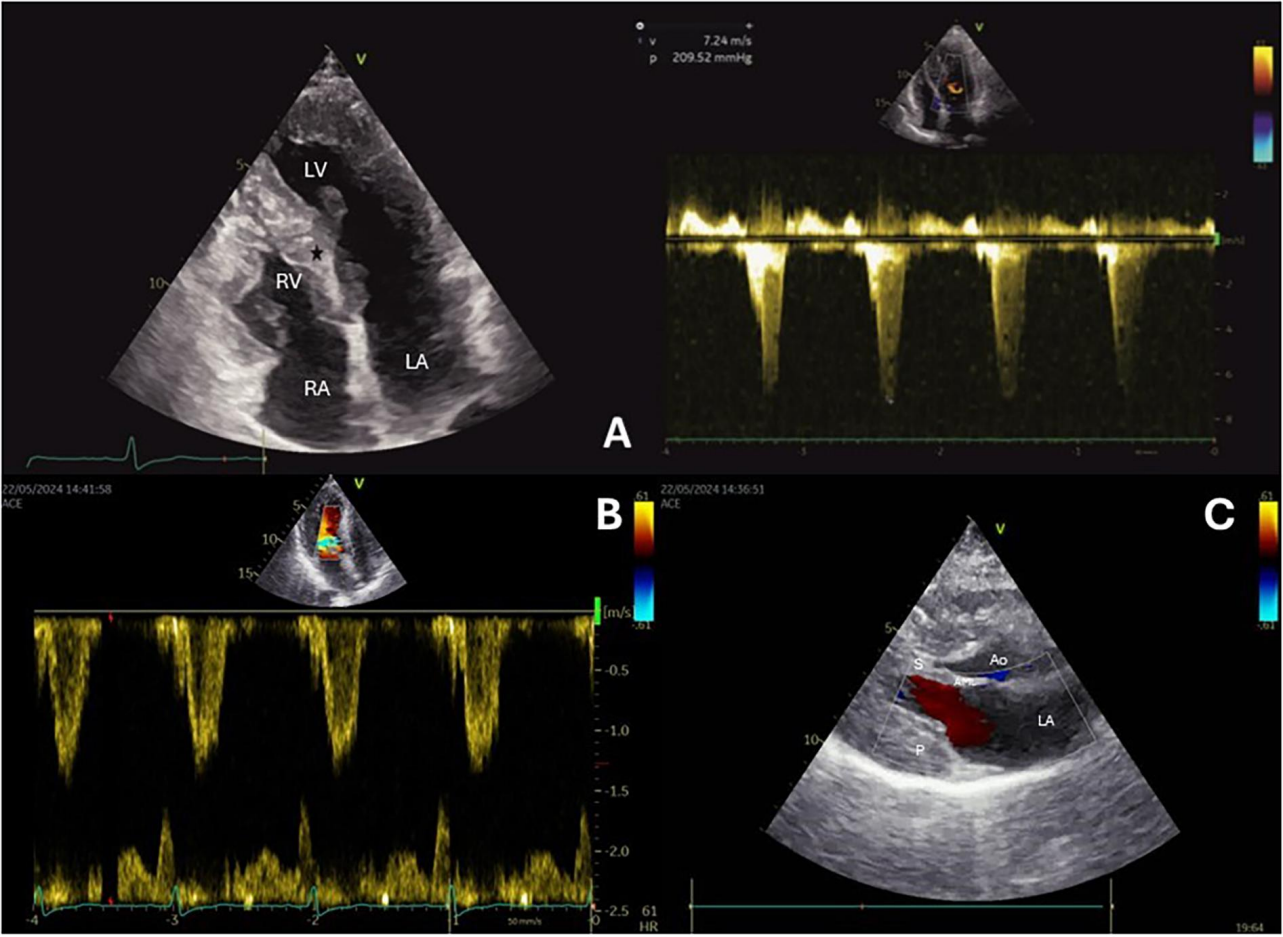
Echocardiography before the procedure (A) shows a hypertrophic basal segment of the interventricular septum (black star), and the continuous wave Doppler signal demonstrates increased LVOT velocity and gradient. After the procedure (B), echocardiography shows reduced interventricular septal thickness, and color Doppler reveals decreased velocity in the LVOT. Color Doppler echocardiography (C) reveals absence of mitral regurgitation.
